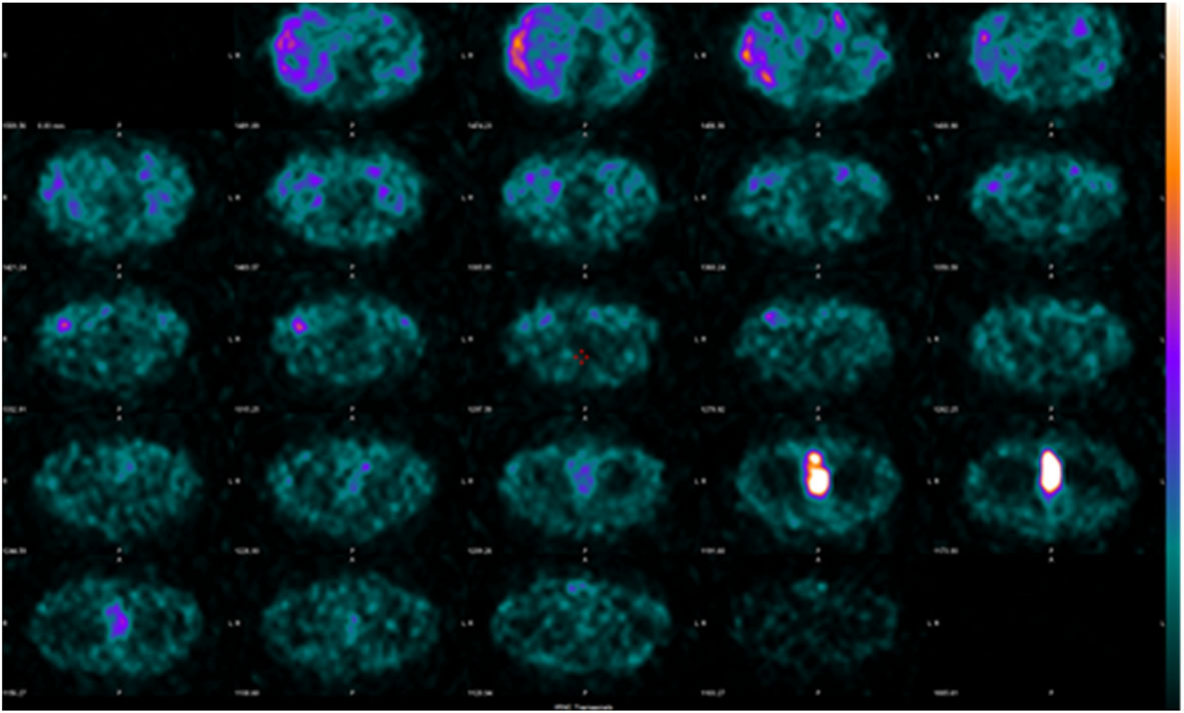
Metaiodobenzylguanidine scintigraphy
